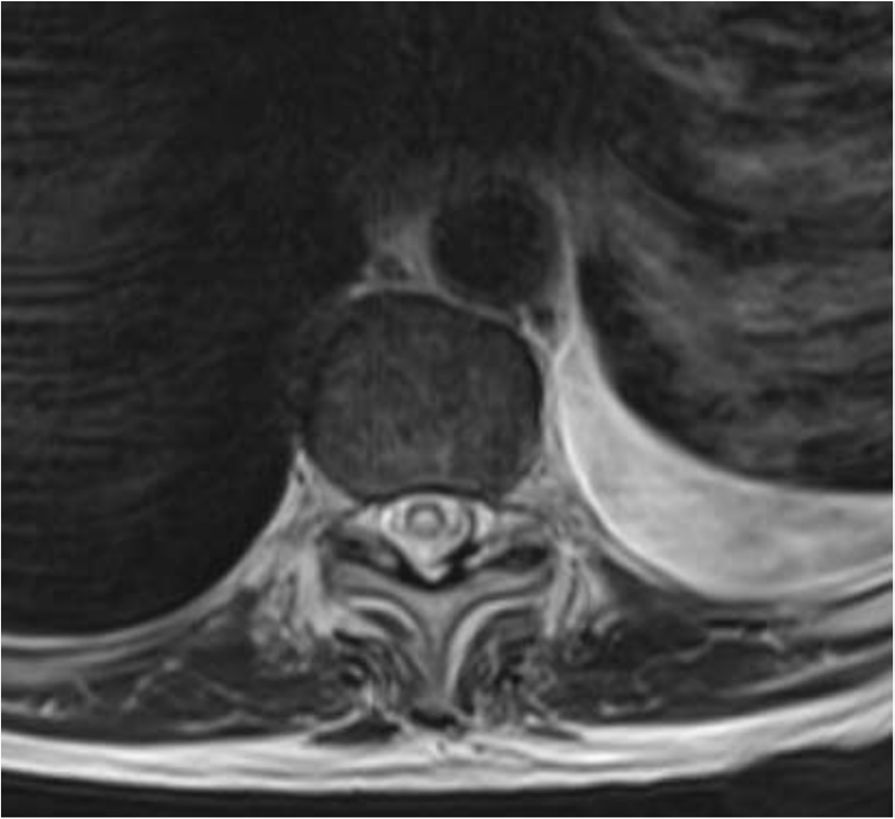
MRI thoracic spine, axial T2: showed central intra medullary T2 hyper intense signal
